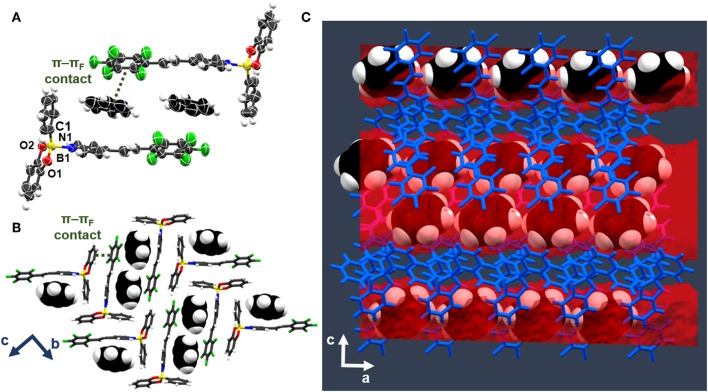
X-ray structure be-pf-sbz⊃ben: (A) stacking involving T-shaped adducts be-pf-sbz⊃ben, (B) p-pF, C-H···p, and van der Waals interactions in bc-plane, and (C) channel formation along a-axis.
